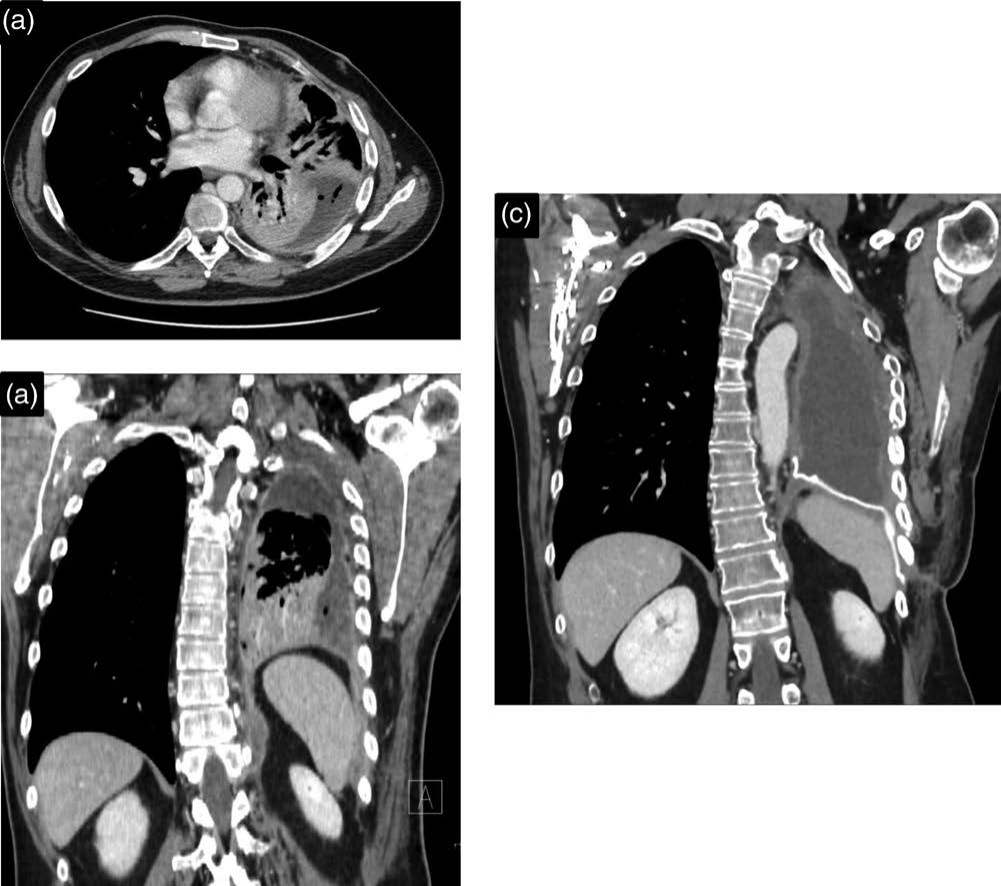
Preoperative contrast-enhanced CT scan images on axial and coronal planes (a) and postoperative contrast-enhanced CT scan image on coronal plane (b).
